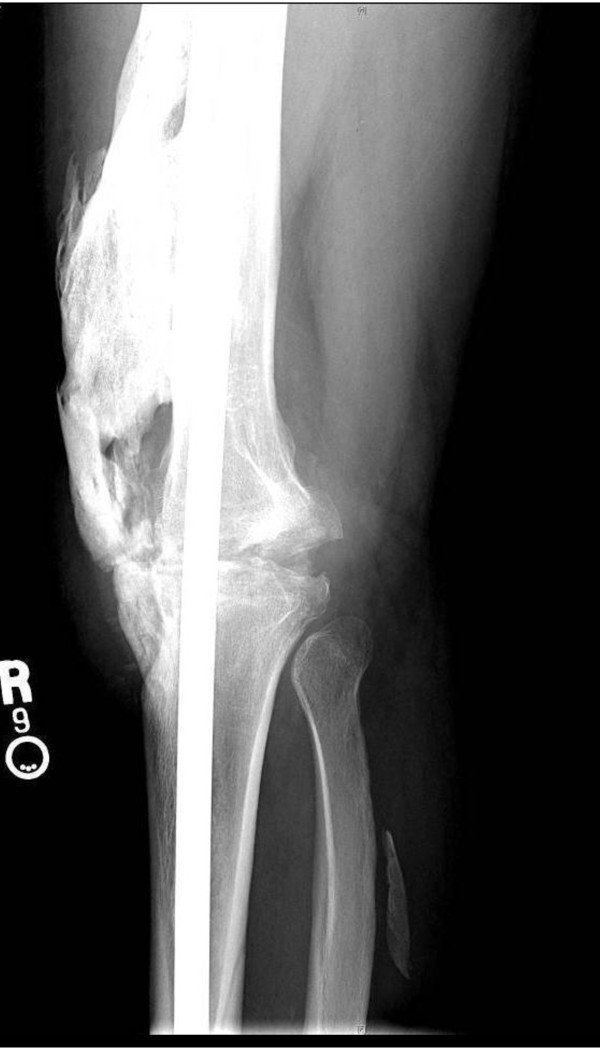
Lateral X-ray of the patient's right knee after surgery to accomplish arthrodesis.
